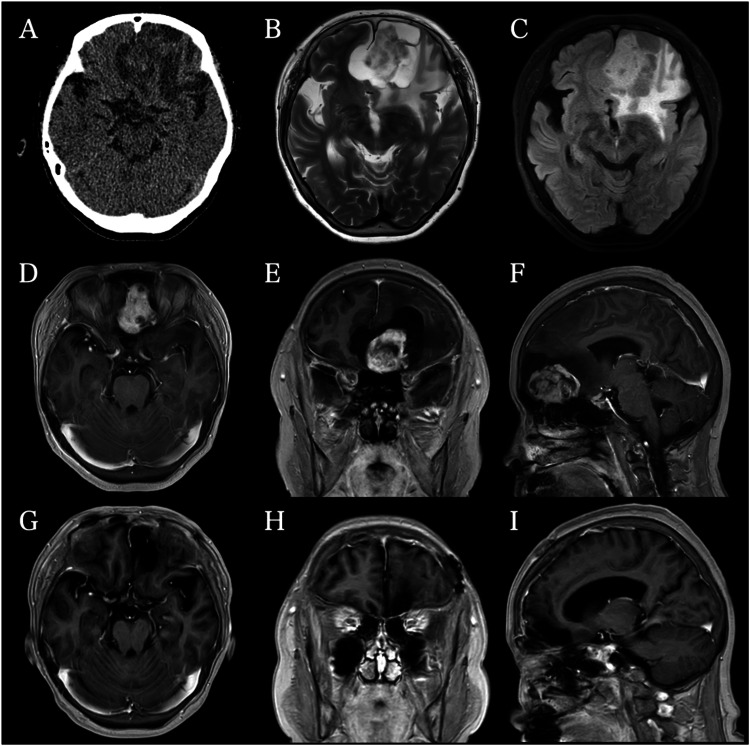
Radiographic Findings of an Olfactory Hybrid Neurofibroma/Schwannoma Preoperative imaging. (A) Axial CT demonstrating the mass lesion in the left anterior cranial fossa. (B, C) Axial T2-weighted and FLAIR MRI images depicting the large extra-axial tumor with peritumoral cysts and perifocal edema.  (D: axial, E: coronal, F: sagittal) On gadolinium contrast, the lesion demonstrated lobulated and heterogeneous enhancement, which seemed to be attached to the left cribriform plate. (G: axial, H: coronal, I: sagittal) Follow-up MRI one year after surgery demonstrated no recurrence.
